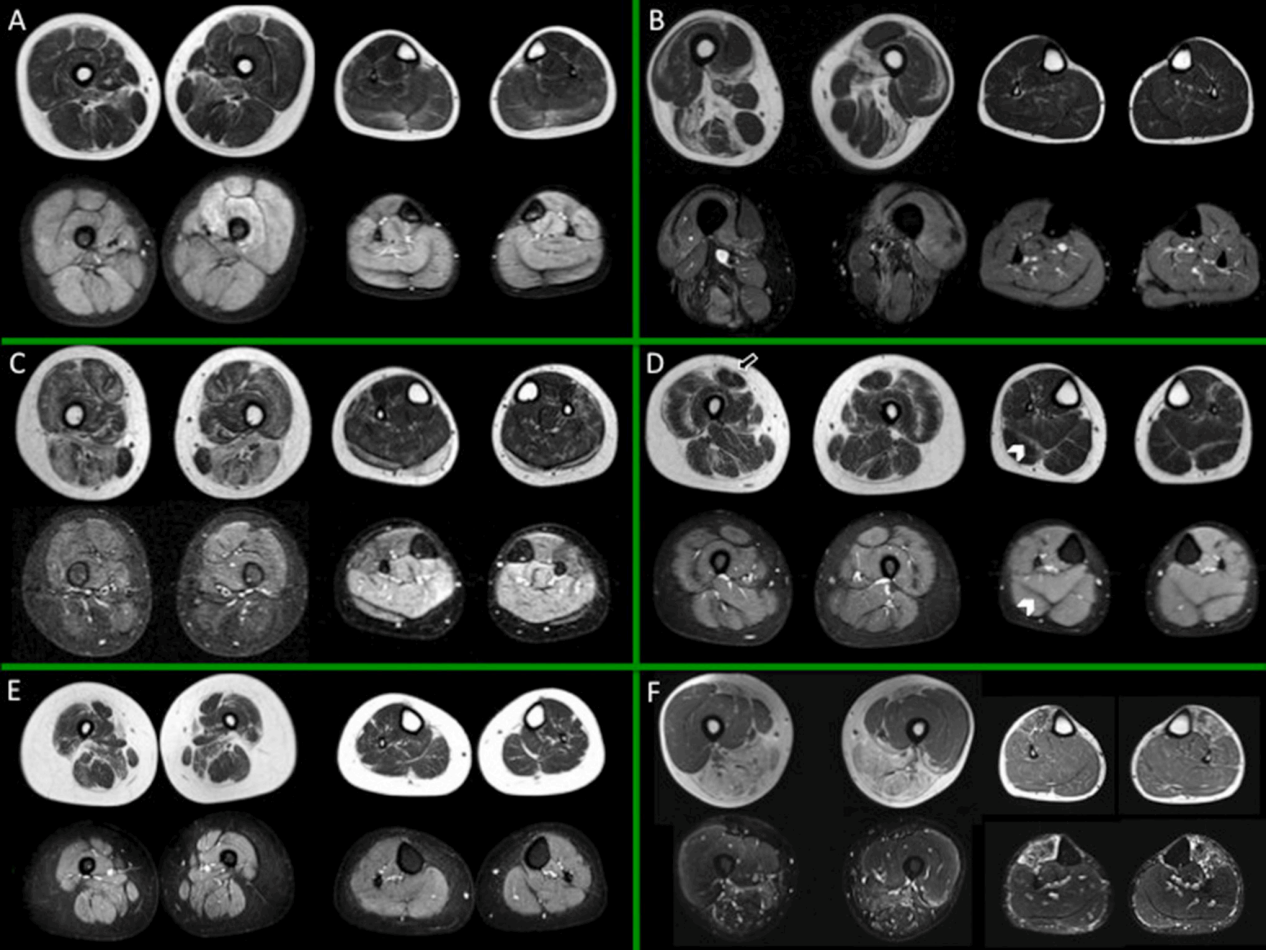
Muscle MRI in a subset of dystrophies and congenital myopathies. For each box, thighs are displayed on the left, legs on the right side; spin-echo T1-weighted images are on top, short-tau inversion recovery (STIR) images on the bottom. (A) Child with Duchenne dystrophy (DMD) showing prominent fat replacement of gemini and adductor magnus and, to a lesser extent, of soleus, peroneal muscles, and rectus femoris bilaterally. Of note, edema of the left quadriceps and, more diffuse, of the leg muscles is evident. (B) Adult subject with Pompe Disease, showing selective fatty replacement of the posterior thigh and relative sparing of leg muscles. Slight patchy STIR hyperintensities are evident bilaterally, mainly involving the thigh musculature, as reported in literature (58). (C) Young subject with beta sarcoglycanopathy, showing important involvement of adductor magnus and longus and harmstring; at leg level the extensor digitorum longus is the most affected muscle. Unspecific slight STIR hyperintensity of the residual calf muscles is evident. (D) Young subject with Bethlem myopathy showing predominantly peripheral involvement both at the level of the thigh and leg muscles. Note the central shadow in the rectus femoris (arrow) and the bat-wing sign in the posterior leg musculature (arrowheads). No edema is evident. (E) Young subject with congenital myopathy due to selenoprotein 1 (SEPN1) showing diffuse muscular atrophy and selective fatty replacement of sartorius and, to a lesser extent, of adductor magnus; no edema is evident. (F) Young subject with facio-scapulo-humeral dystrophy (FSHD), showing involvement of the posterior thigh muscles and of tibialis anterior (TA), with sparing of the quadriceps bilaterally, but with rectus femoris involvement. Edema is evident at the level of TA and harmstring muscles and, peripherally, of vastus lateralis. All presented MRI images have been collected during routine patient care.
